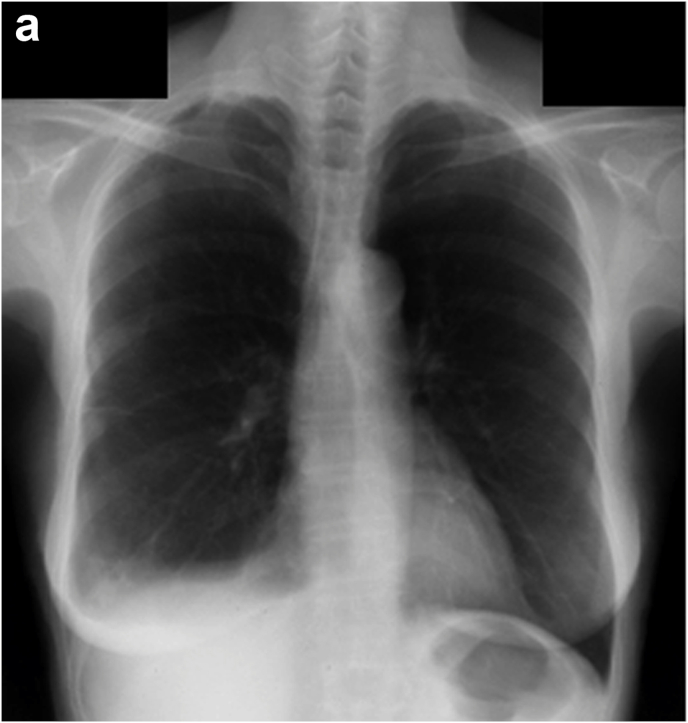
Chest radiographs July 2006 The right costophrenic angle is blunted, but no other abnormalities were noted at this point.
